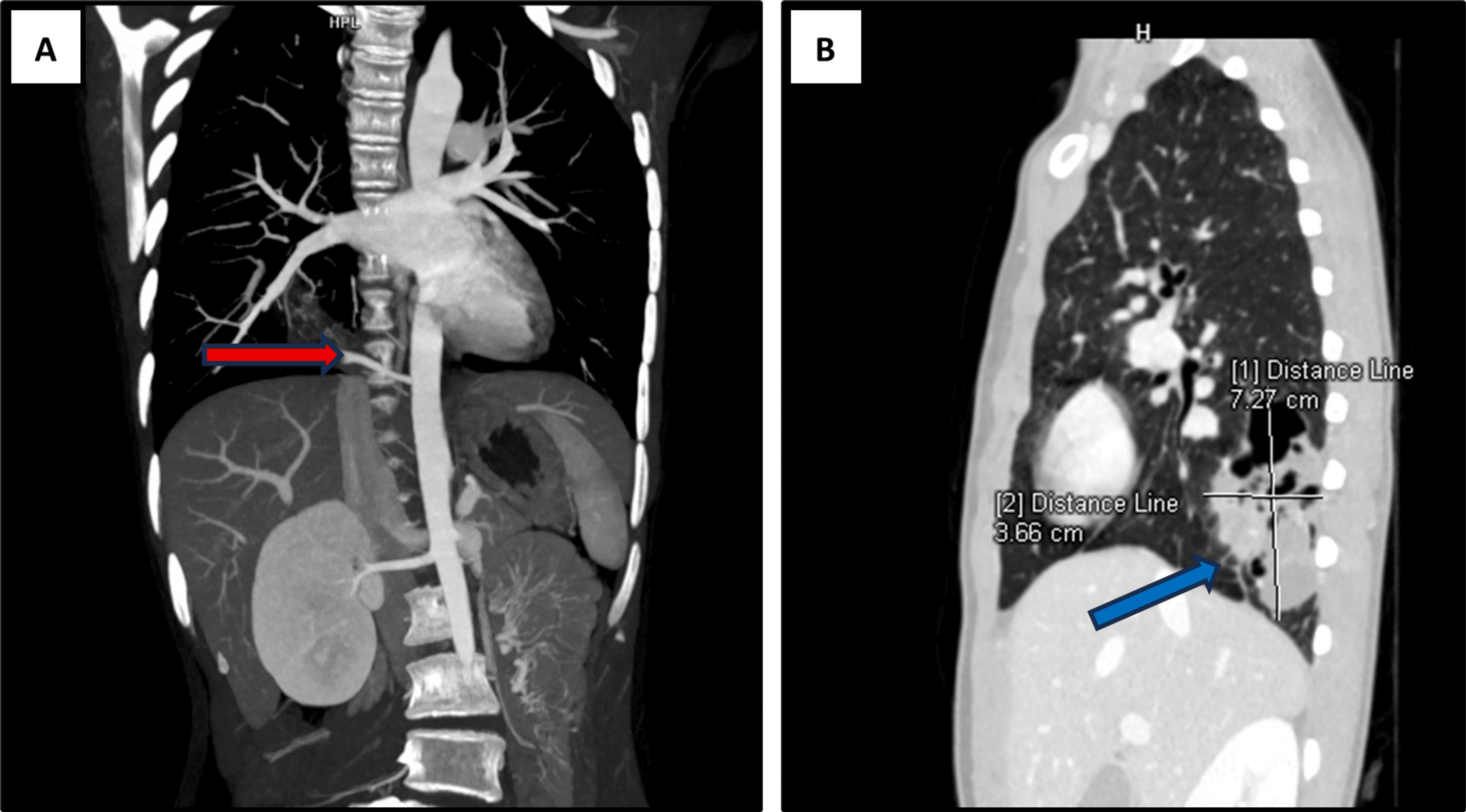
(A) CT angiographic image of the coronal section of the chest and abdomen demonstrating an anomalous systemic arterial supply (red arrow) arising from the descending thoracic aorta and coursing towards a well-defined lesion in the posterior basal segment of the right lower lobe. (B) Sagittal section of the lung window of CECT of the chest showing the sequestrated lung segment (blue arrow) measuring approximately 7.2×3.6 cm and showing no obvious communication with the tracheobronchial tree CT: computed tomography; CECT: contrast-enhanced computed tomography
